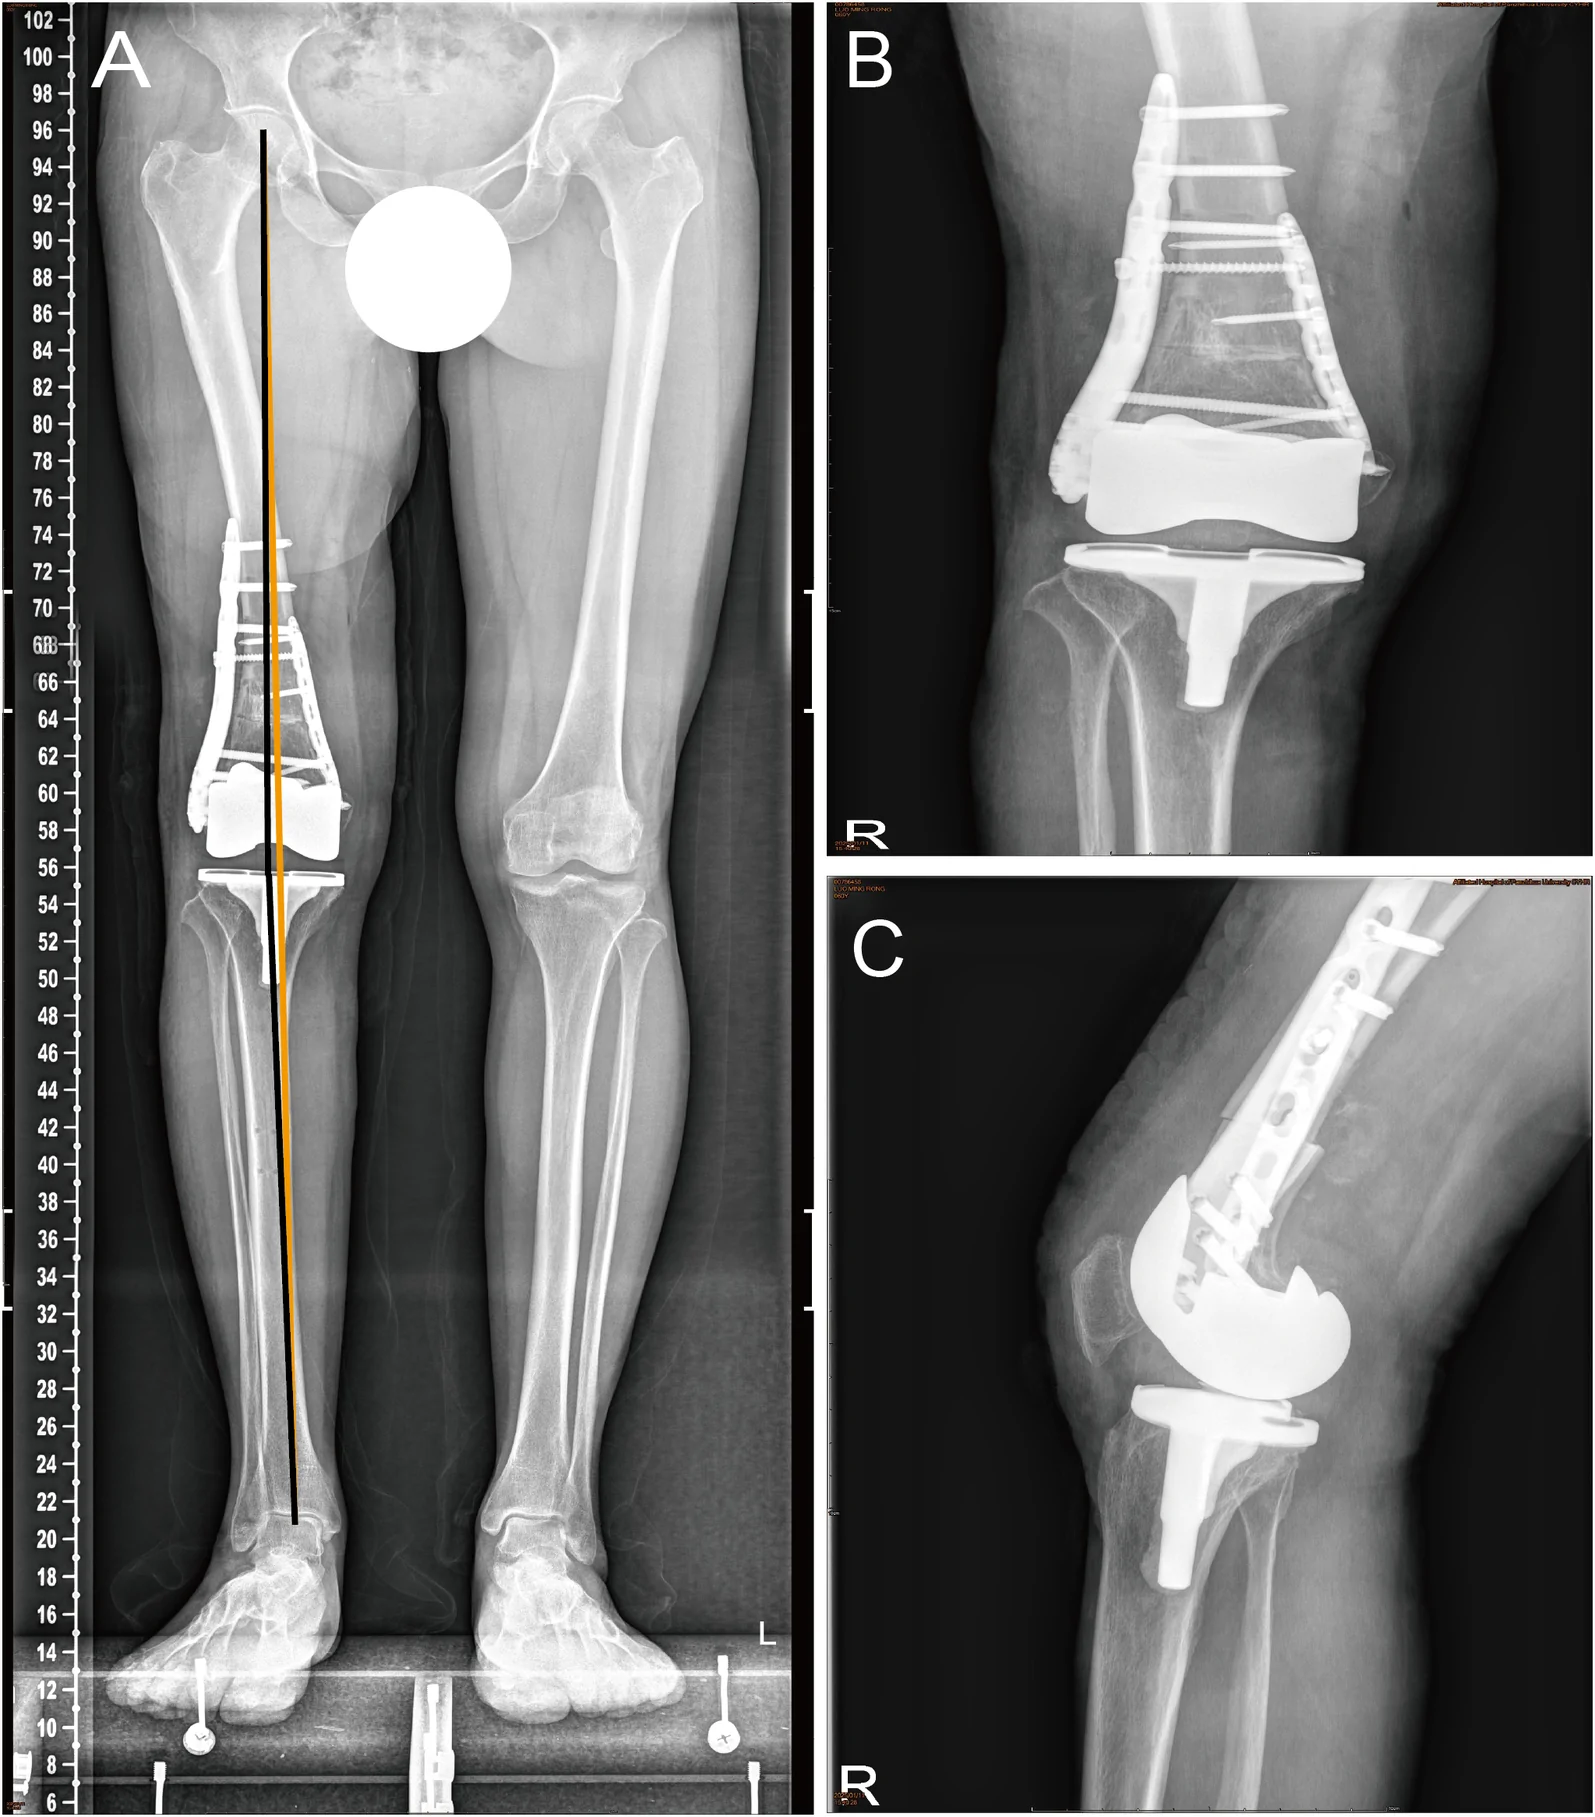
Immediate postoperative radiographs (A) full-length postoperative radiograph showing correction toward near-neutral alignment: HKA improved from 174.4° preoperatively to 178.7° postoperatively (1.3° residual varus), and MAD decreased from 20 mm to 2.5 mm medial to the knee center. The TKA components and fixation hardware are satisfactorily positioned. (B,C) Anteroposterior and lateral radiographs showing correction of the femoral antecurvatum and satisfactory apposition of the osteotomy surfaces.
